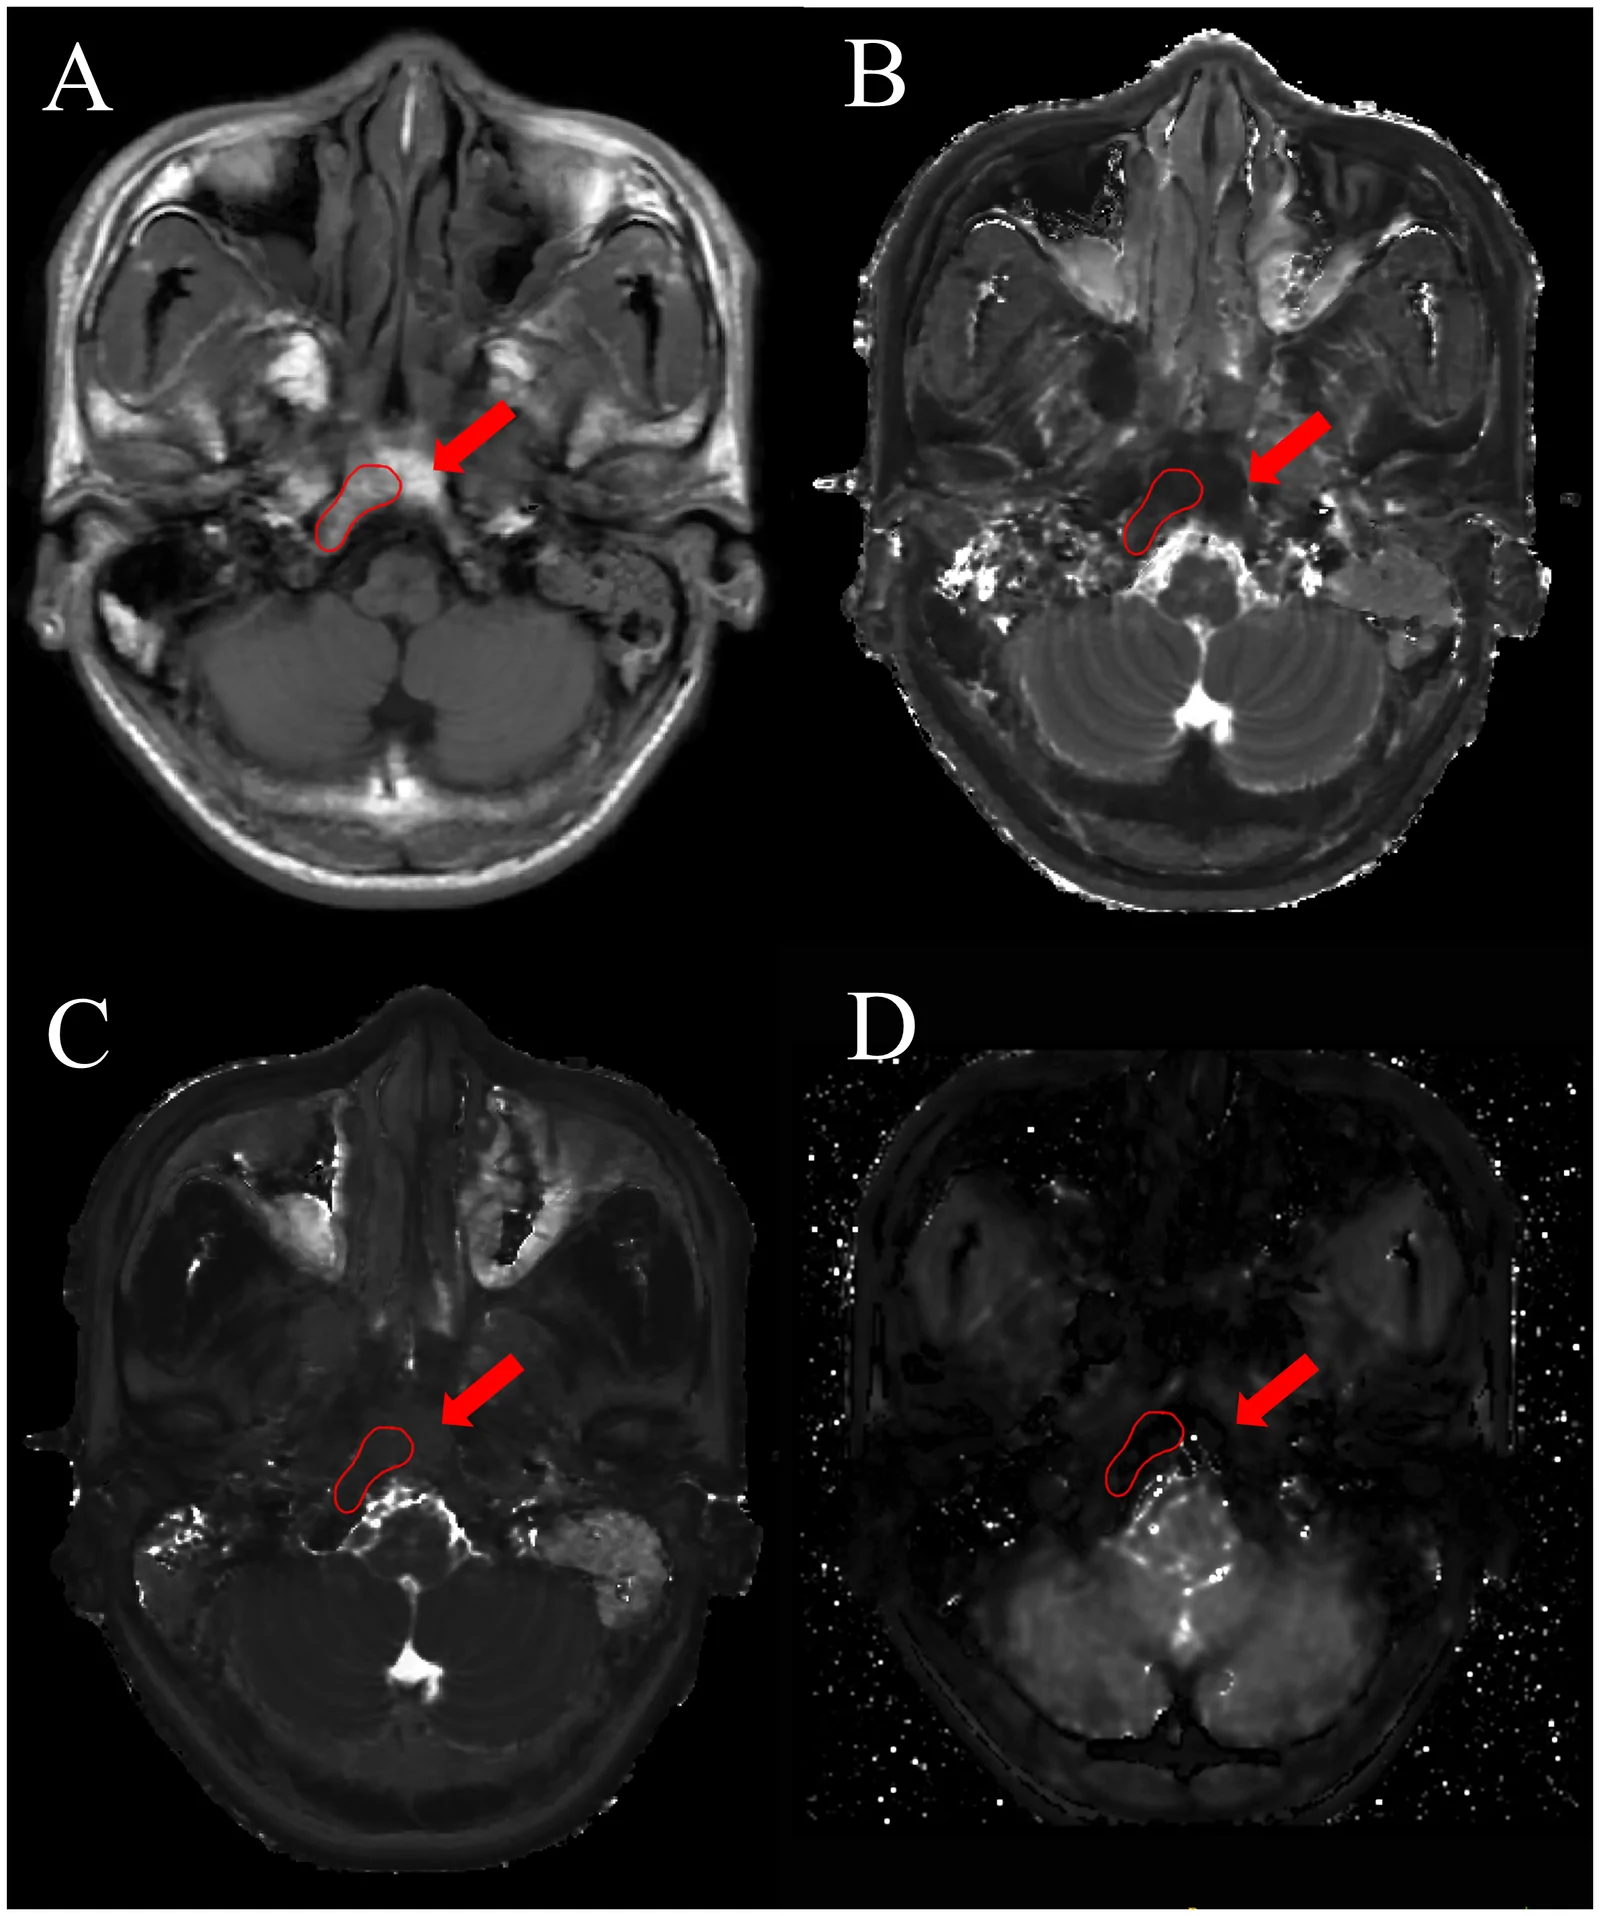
Representative images of clival invasion in a 34-year-old man with locally advanced nasopharyngeal carcinoma. The volume of interest (VOI; red outline) was manually delineated on conventional T1-weighted imaging (A) to encompass the tumor lesion within the clivus (red arrow). The same VOI was subsequently copied and applied to the corresponding co-registered quantitative T1 map (B), quantitative T2 map (C), and quantitative T2* map (D). Although lesion conspicuity was limited on the T2* map (D), likely because of susceptibility-related artifacts at the skull base, the co-registered VOI enabled consistent quantitative parameter extraction across all maps.
